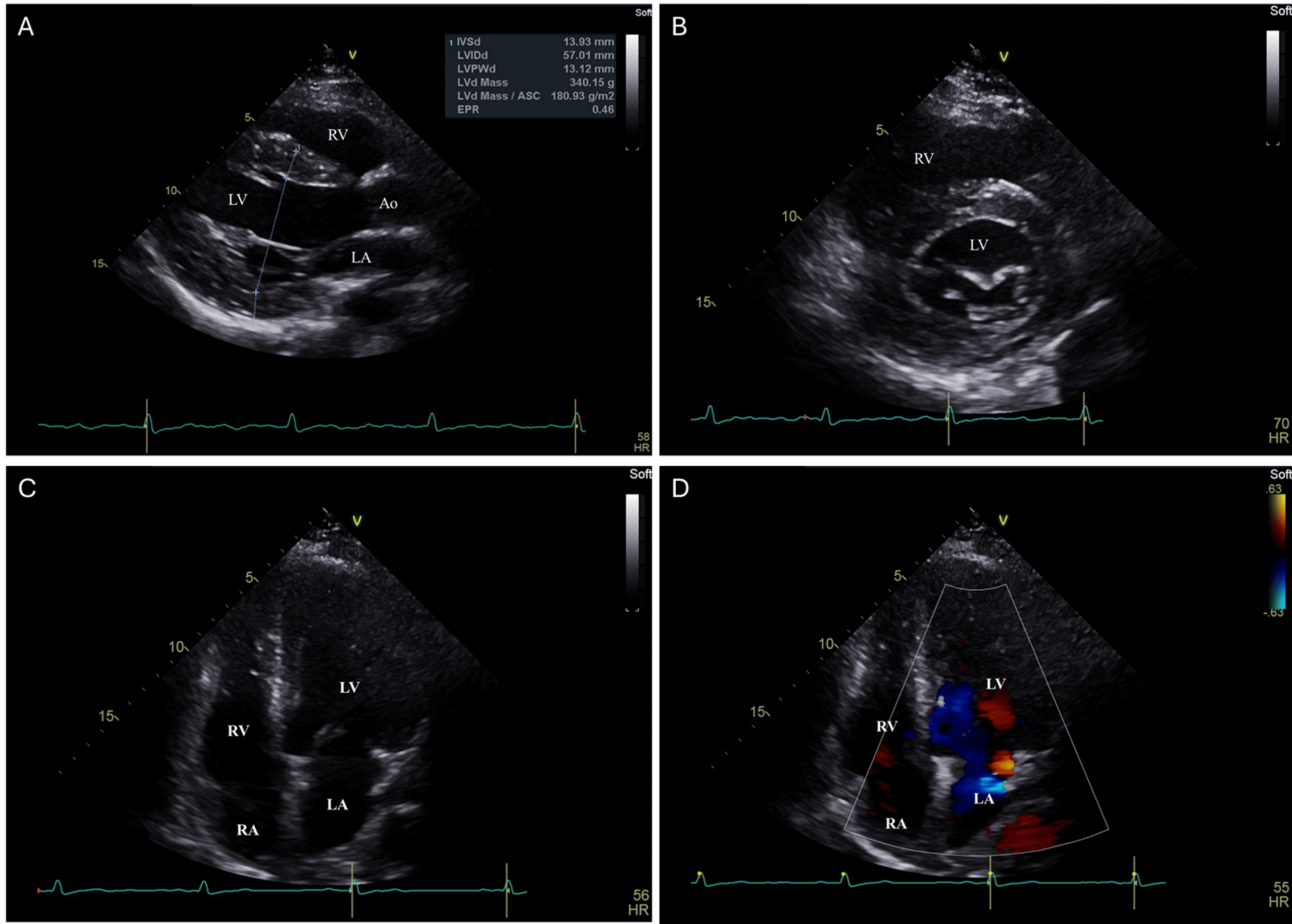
Echocardiographic evaluation demonstrating concentric left ventricular hypertrophy, mild left atrial dilation, and mild mitral regurgitation. A. The parasternal long-axis view showed increased thickness of the interventricular septum and posterior left ventricular wall (diastolic left ventricular posterior wall diameter: 13.12 mm), with a relatively small ventricular cavity, consistent with concentric left ventricular hypertrophy. B. Mid-ventricular parasternal short-axis view demonstrated uniform circumferential thickening of the left ventricular walls, compatible with concentric hypertrophy associated with long-standing hypertension. C. The apical four-chamber view revealed a mildly enlarged left atrium (left atrial volume index: 40 mL/m^2^) and increased left ventricular wall thickness. No significant structural abnormalities of the mitral valve were visible in this grayscale view. D. Color Doppler in the apical four-chamber view showed a small posteriorly directed mitral regurgitation jet, consistent with mild regurgitation, with otherwise normal appearance of the mitral leaflets. Ao: aorta; LA: left atrium; LV: left ventricle; RA: right atrium; RV: right ventricle.
